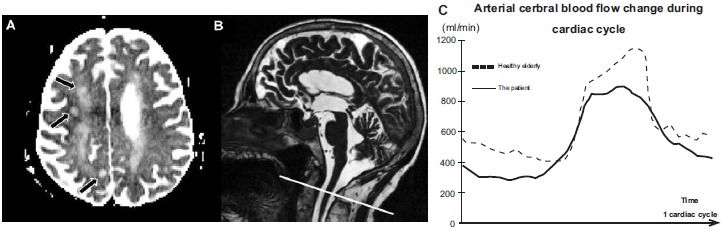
A: The T2* Magnetic Resonance Imaging shows three areas of semi recent ischemia in the white matter, including: frontal right posterior, right posterior transitional zone and beside the left central sulcus.
